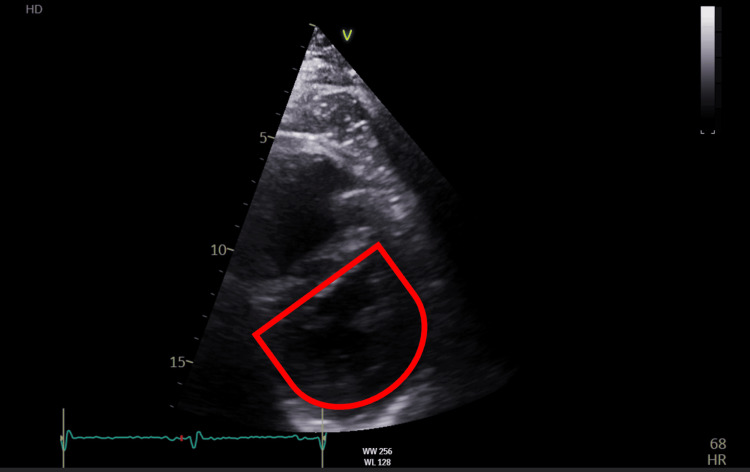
Echocardiogram shows diastolic interventricular septal flattening a sign of right ventricular volume overload - 'D' Shape
